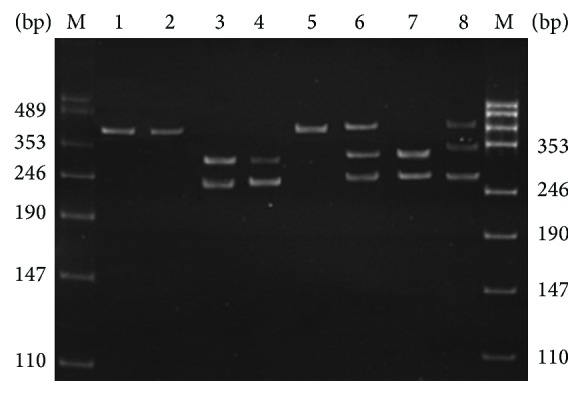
Electrophoresis of amplification fragments of the MMP9 promoter region for the detection of the −1562C/T polymorphism. Lanes 1, 2, and 5 are the CC genotype; lanes 3, 4, and 7 are the TT genotype; lanes 6 and 8 are the CT genotype. M: DNA molecular weight marker.
